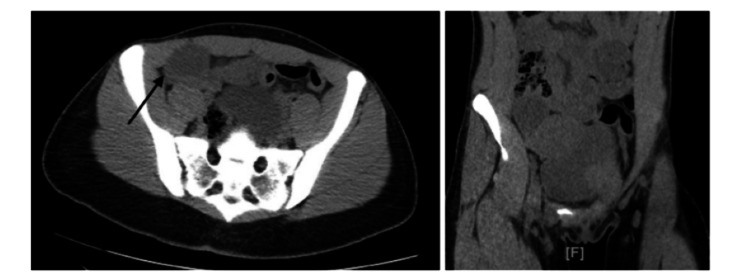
Computed tomography imaging showing the right and left lower quadrant ovarian cysts.
